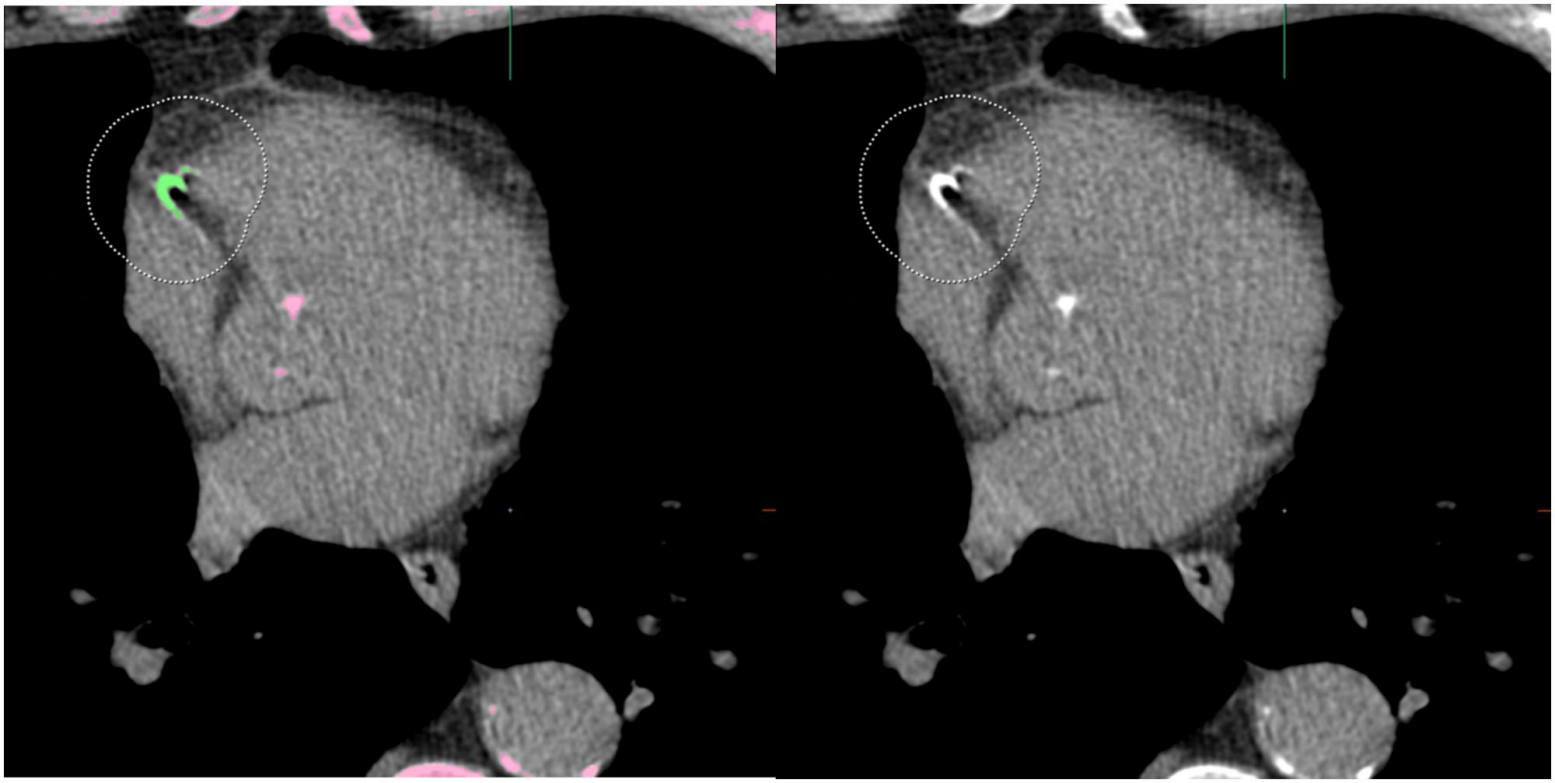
Example of an acquisition error of the coronaries with a 1-run, non-gated, low-dose chest CT part of a lung cancer screening program. The automated artificial intelligence (AI)-based coronary artery calcium (CAC) quantification software segmented a calcification in the right coronary artery (RCA) (segmentation in green), which has a motion artifact making the calcification seem larger, consequently overestimating the calcium.
